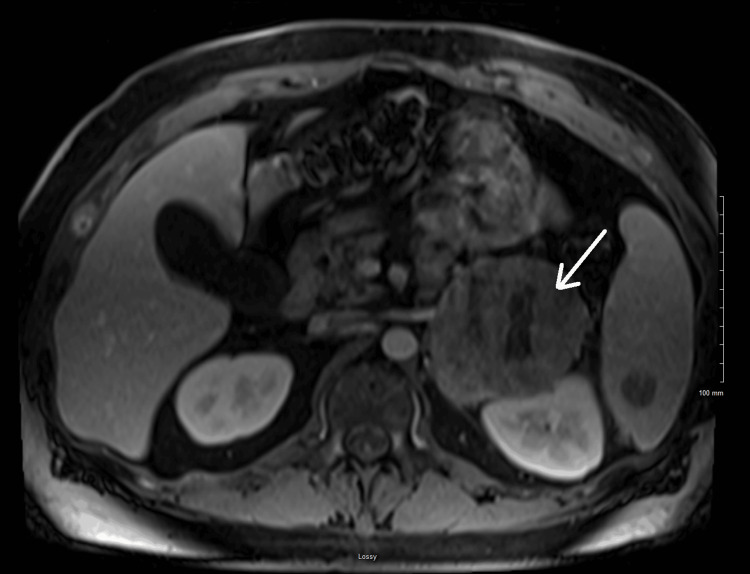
MRI image of the left adrenal mass MRI: Magnetic resonance imaging
